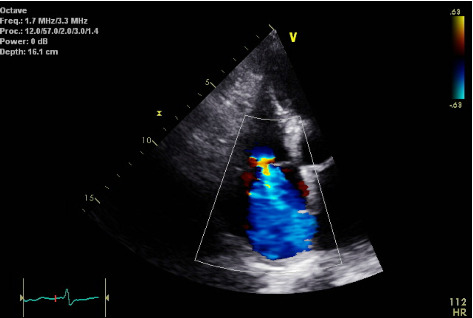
Color Doppler echocardiographic image showing turbulent regurgitant jet across the tricuspid valve with a regurgitant velocity of 264 cm/s and an assumed right atrial pressure of 8 mmHg. These findings are suggestive of severe tricuspid regurgitation.
